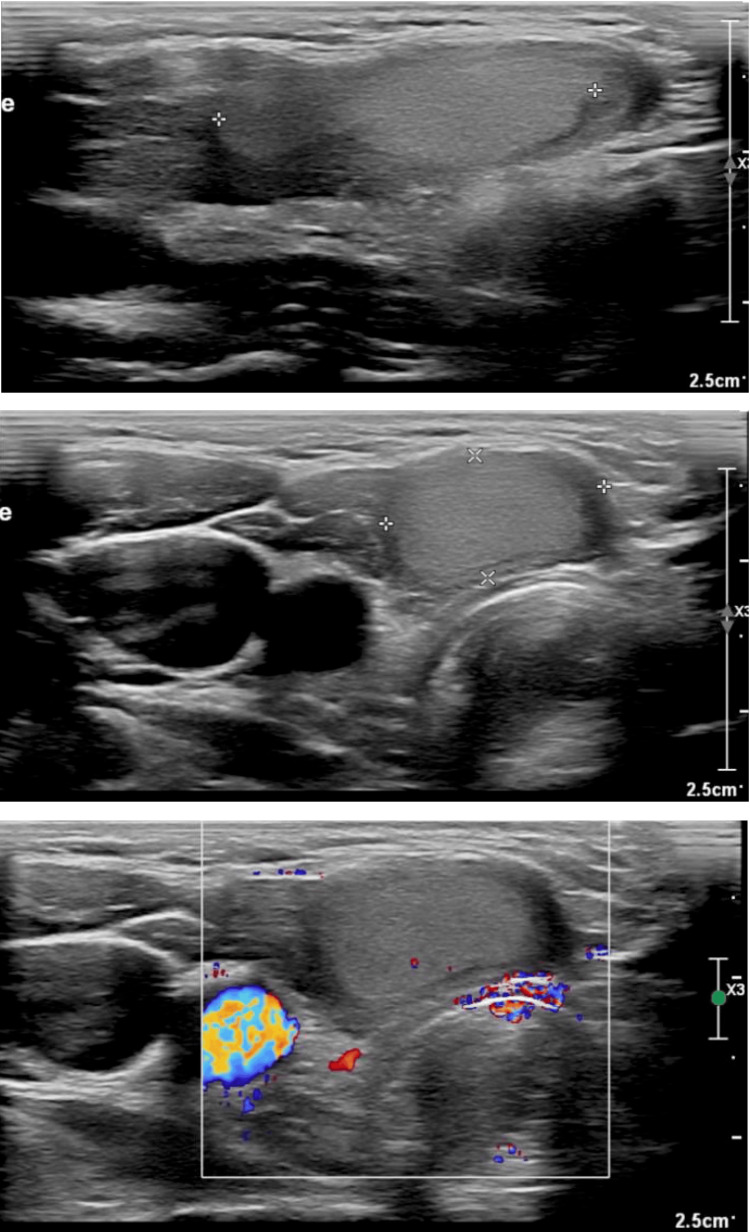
Ultrasound of the pre-tracheal lesion showing an elongated, well-defined isoechoic lesion measuring 2.5 x 1.5 x 0.8 cm in the operative bed The lesion showed no calcifications
